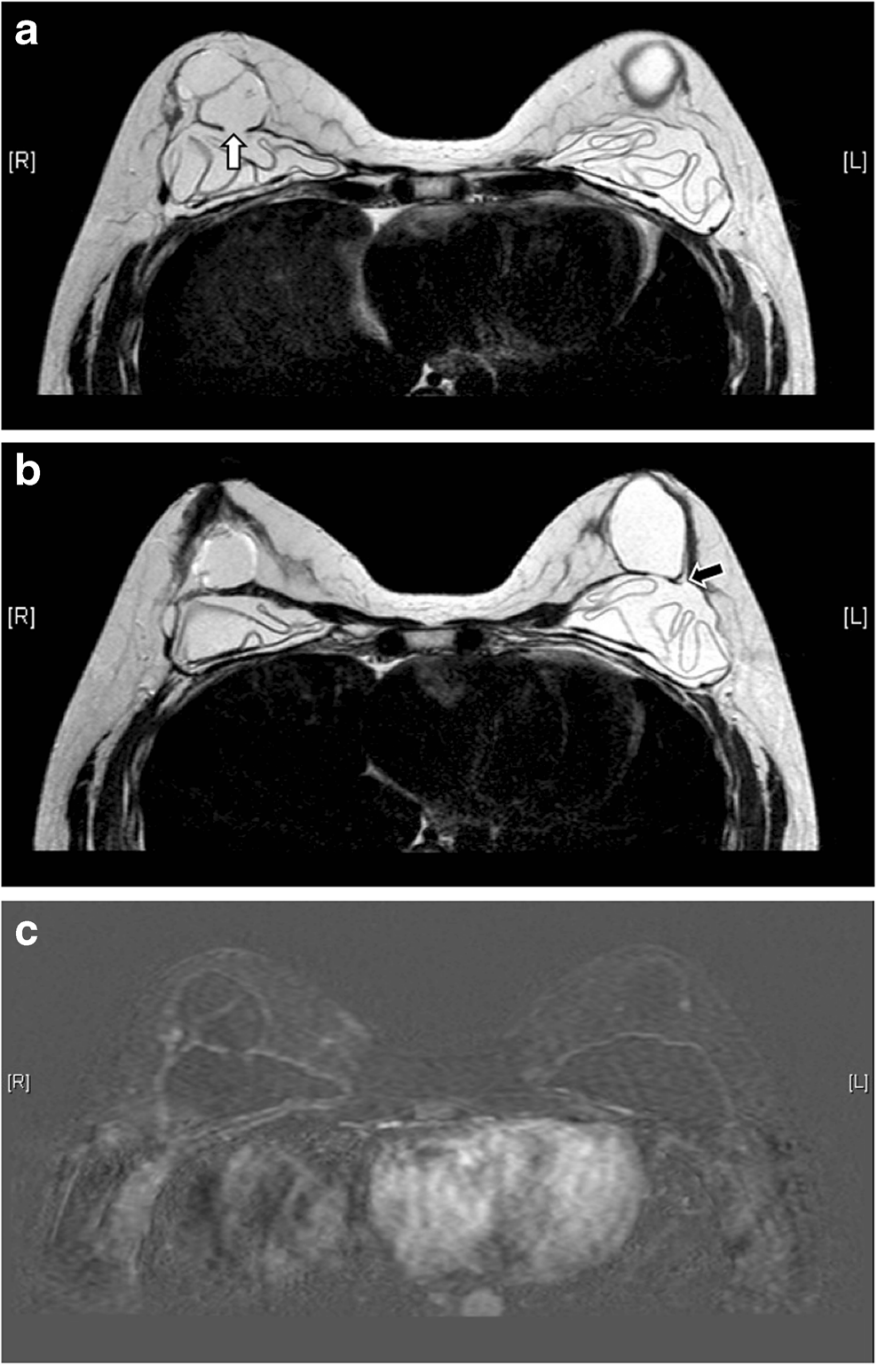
Extracapsular rupture. T2-weighted axial MR images (a, b) show bilateral extracapsular ruptures. Defects are seen at bilateral fibrous capsules (white arrow on the right and black arrow on the left). Both collapsed implants demonstrate the linguine sign. On the T1-weighted post-contrast image with subtraction (c) there is a thin reactive rim of enhancement surrounding the collapsed implants
